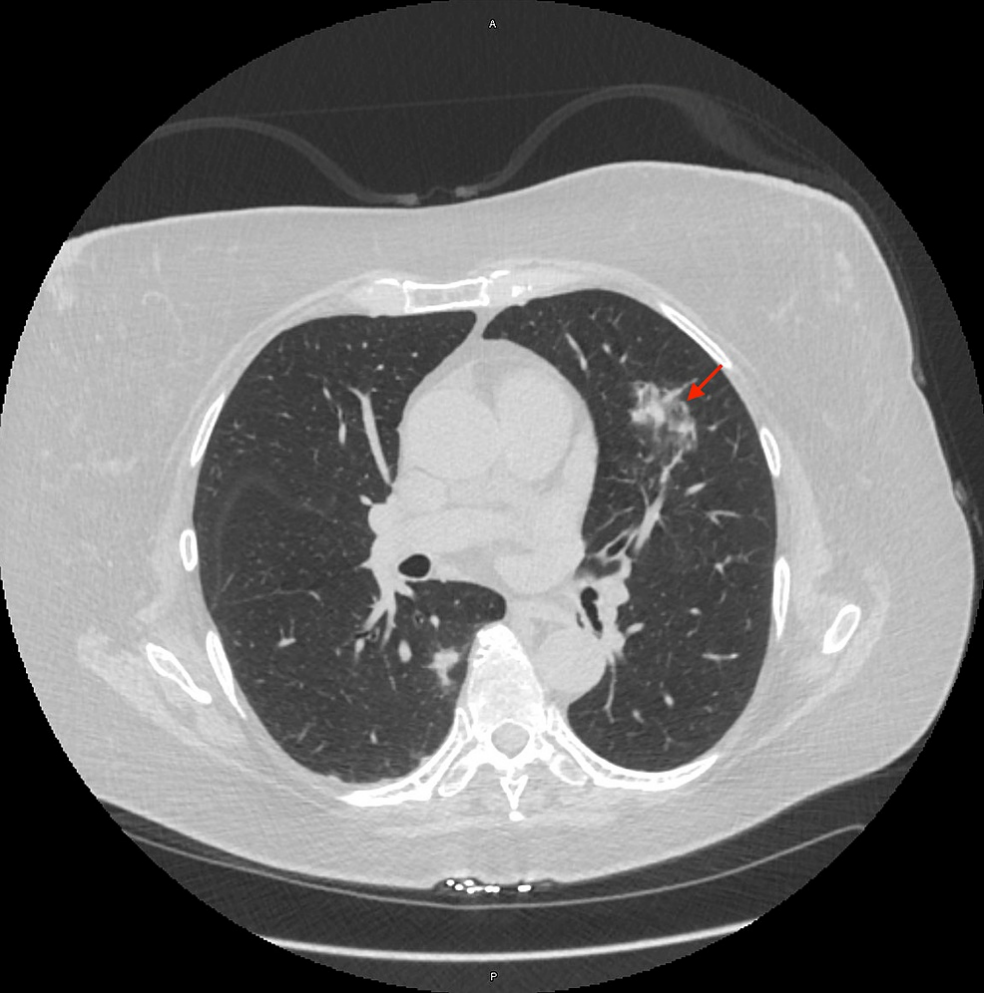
CT chest Red arrow - Superior lingular lesion
